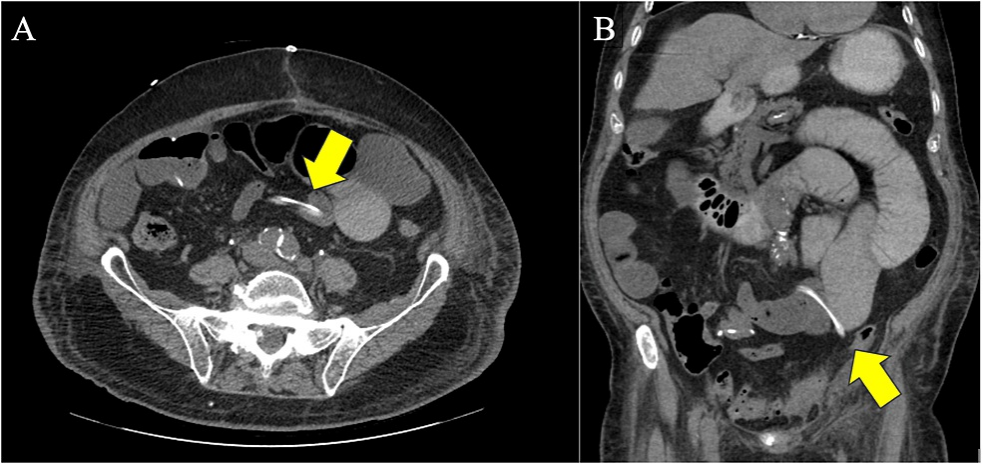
CTAP without contrast on post-op day 7, indicating a transition point at the LLQ with mild compression from the percutaneous surgical drain (A: Axial, B: Coronal). CTAP: CT Abdomen and Pelvis; LLQ: Left lower quadrant.
